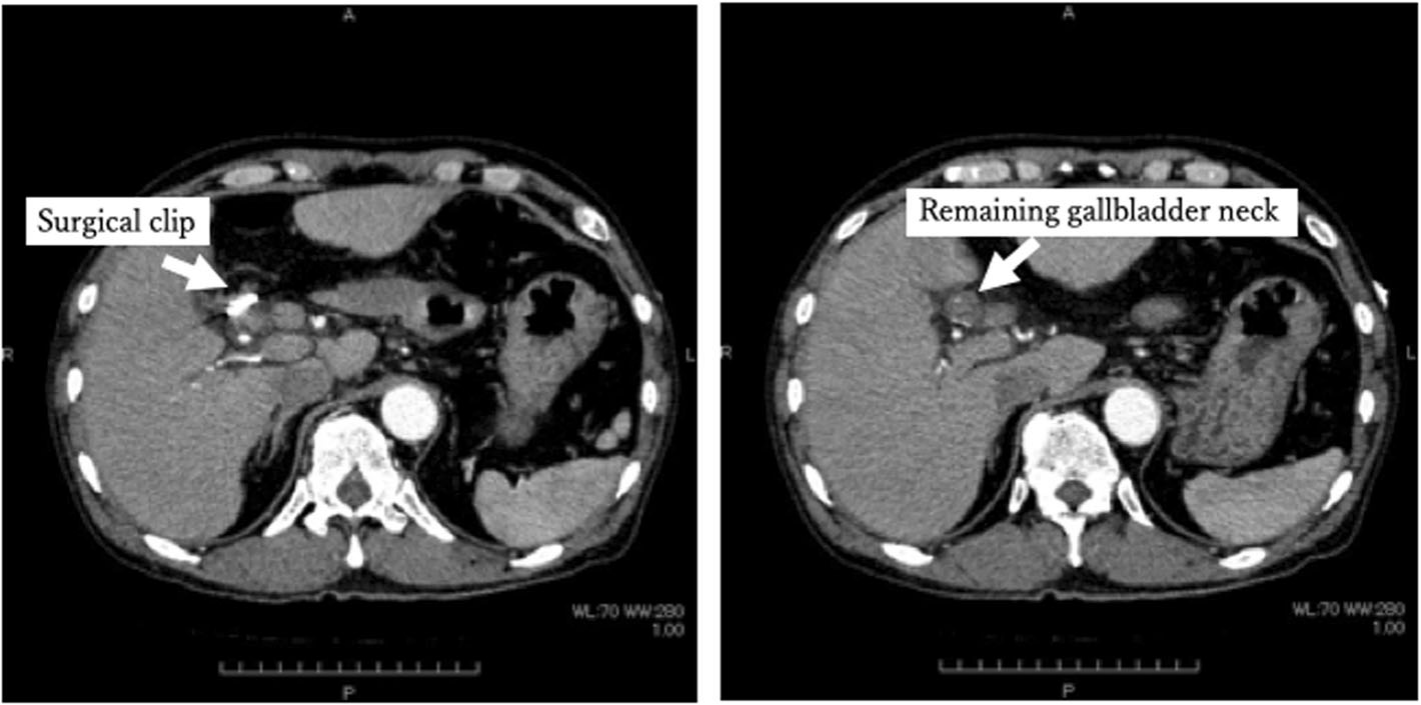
Contrast CT after LC. Contrast CT showed no leakage of the contrast agent and pseudoaneurysm formation
